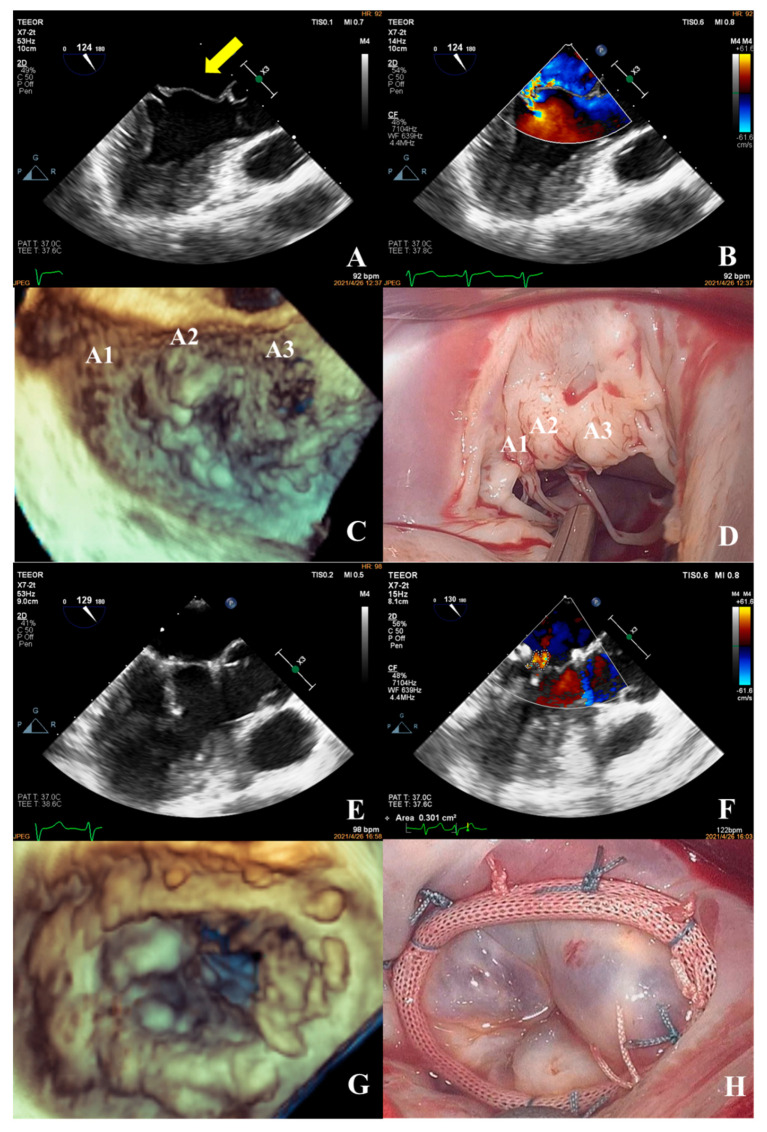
Transesophageal echocardiography was performed; the anterior mitral valve leaflet prolapsed into the left atrium in diastole (A) (yellow arrow shows prolapse of the anterior leaflet) and a severe eccentric regurgitant signal was seen in systole (VC: 5.5 mm) (B). Prolapse of the mitral valve in the anterior leaflet A1, A2, and A3 segments was observed in 3D images (C). Intraoperative confirmation of prolapse in the A1, A2, and A3 segments of the anterior mitral valve leaflet (D) was consistent with the preoperative diagnosis. Intraoperatively, two artificial chordae were reconstructed and a mitral valvuloplasty ring was implanted. After resuscitation, the result of the repair was good, with a height of coaptation of 6.4 mm (E), and a tiny regurgitant signal was observed in diastole, with a regurgitant area of 0.3 cm^2^ (F). The annuloplasty ring of the valve was visible on 3D ultrasound (G), which was consistent with the intraoperative view (H).
